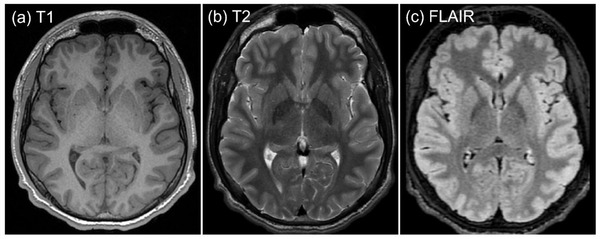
(a) T1‐weighted, (b) T2‐weighted, and (c) T2 FLAIR MRI sequences acquired on a healthy 17‐year‐old male, representing key sequences of a clinical MRI protocol
